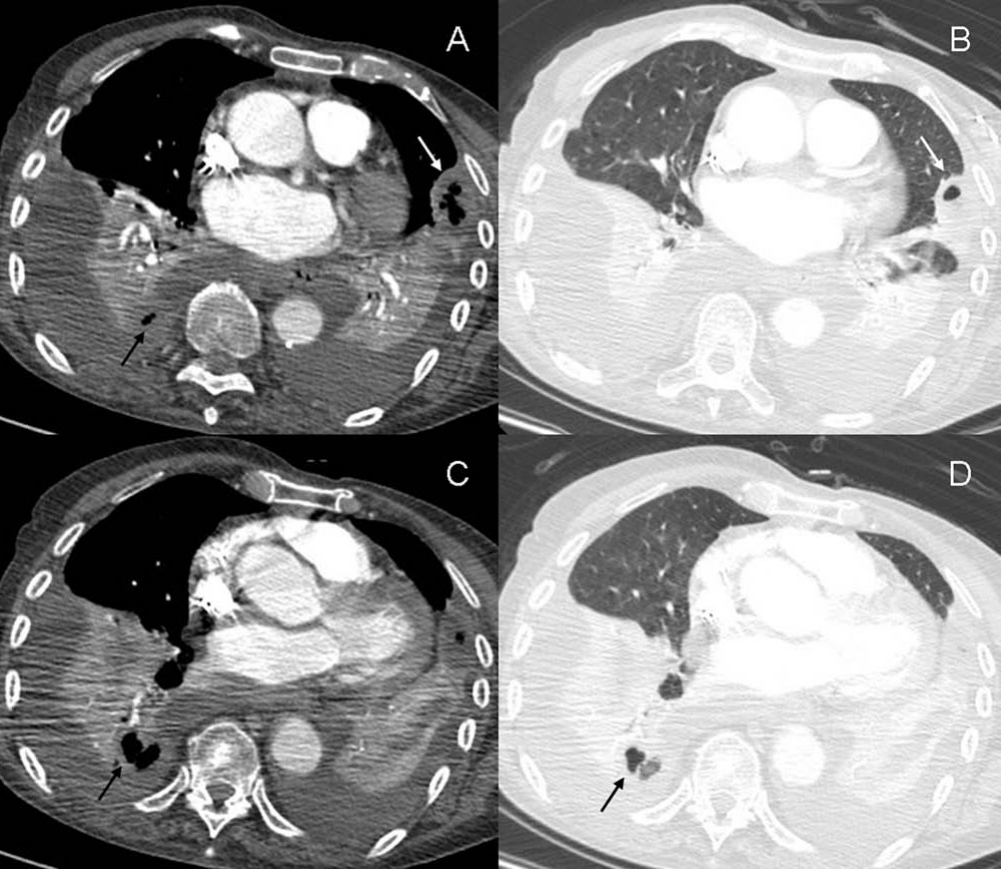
Contrast-enhanced multi-slice CT of the thorax performed 48 hours after admission. Multi-slice CT of the thorax confirmed presence of bilateral pleural effusion and consolidation of lower lobes and lingula; cavitation was revealed in the pleural-based consolidation of the lingula (Figure 2A-B, white arrows) and in the right lower lobe (Figure 2A-C-D, black arrows). These findings were considered indicative of a necrotizing, gangrenous pneumonia.
